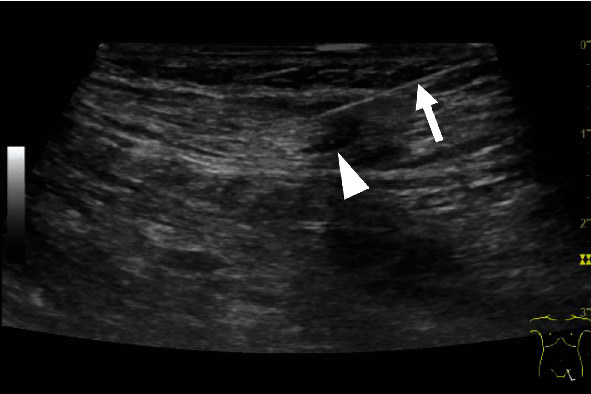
Ultrasonography performed during intranodal lymphangiography demonstrating a 23-gauge needle (arrow) being advanced into an inguinal lymph node (arrowhead).
